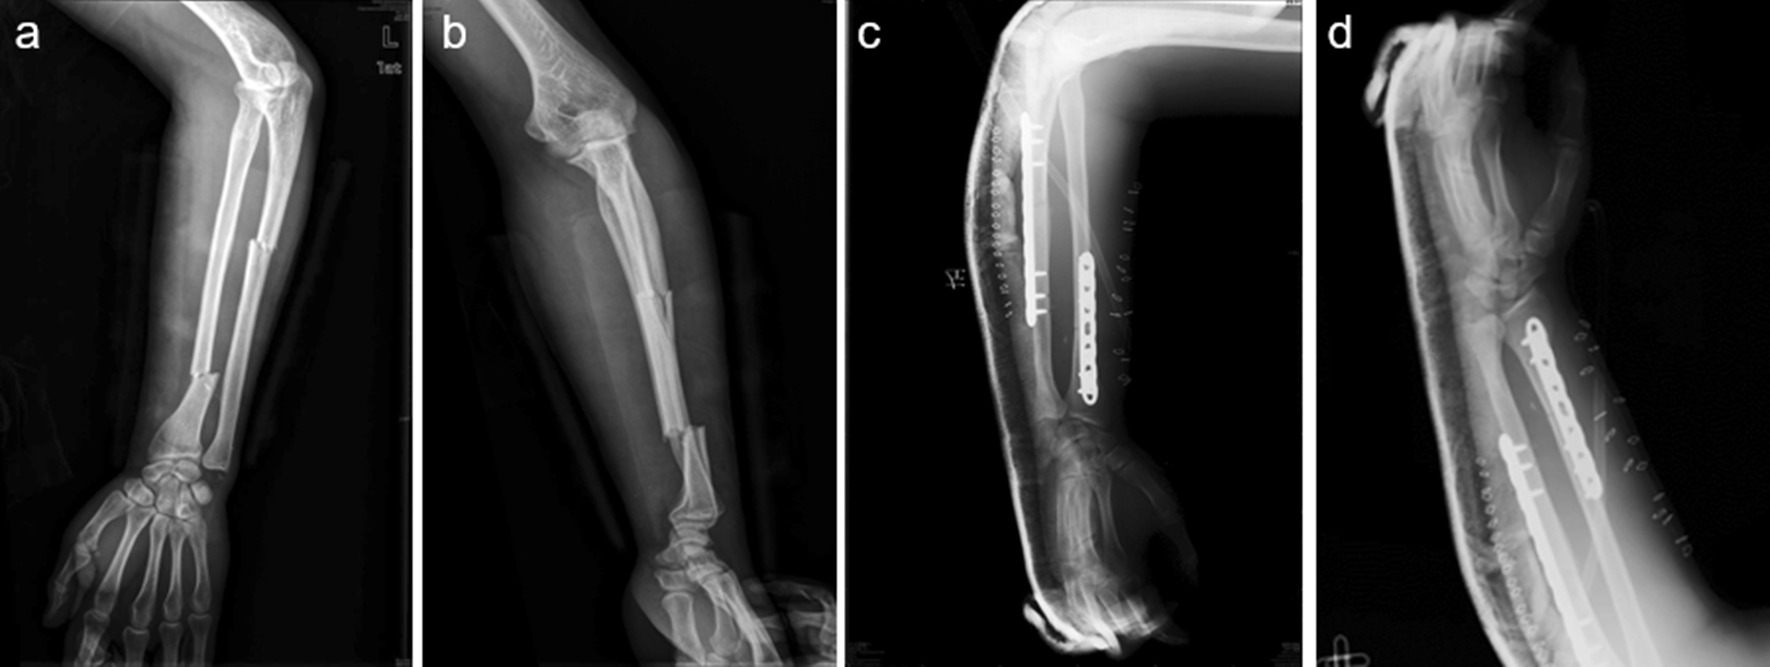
a–b: Preoperative radiographs show a classic Monteggia and Galeazzi fracture, also named “floating radius.” c–d This patient received an ORIF and plaster fixation with the forearm
